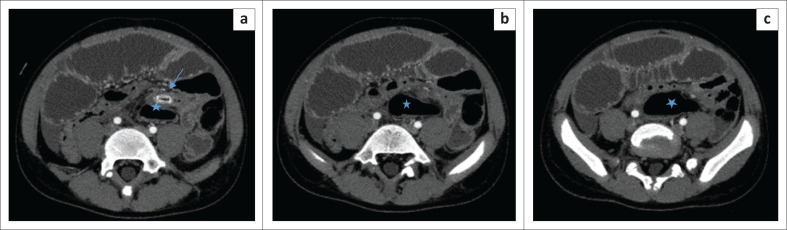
(a-c) (cephalad to caudad): A 12-year-old boy presented with abdominal distension, vomiting and a raised white cell count. Axial CT revealed the abnormal caecal position (blue star) in the left lower quadrant with an anterior cephalad thickened appendix containing an appendicolith (arrow) and adjacent fat stranding.
